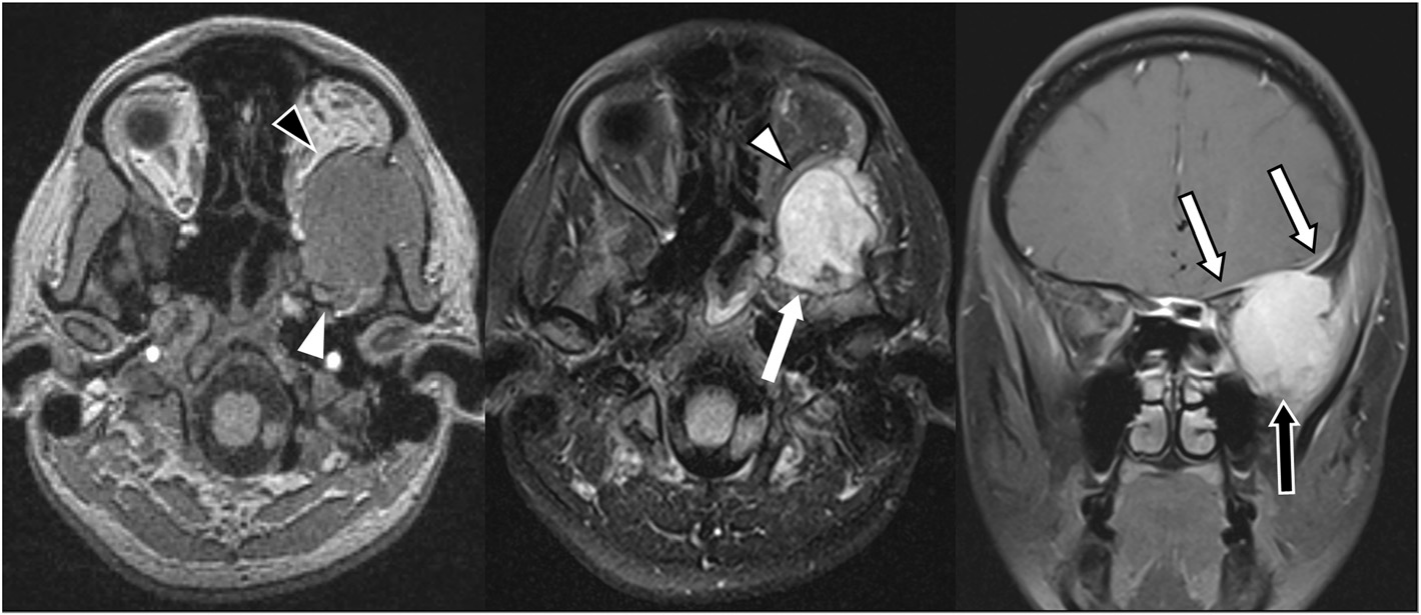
Axial unenhanced T1-weighted magnetic resonance image demonstrating a large mass in the left middle fossa (white arrowhead) extending through the left greater sphenoid wing into the left orbit (outlined black arrowhead). The fat-suppressed axial T1-weighted gadolinium-diethylenetriaminepentaacetic acid (Gd-DTPA)-enhanced image demonstrates the same findings (white arrow, outlined white arrowhead, respectively). The mass is enhanced homogeneously. The coronal fat-suppressed axial T1-weighted Gd-DTPA-enhanced image demonstrates the left middle fossa component (outlined black arrow) and extension up and over the margin of the left sphenoid ridge producing dural thickening and enhancement here (outlined white arrows).
